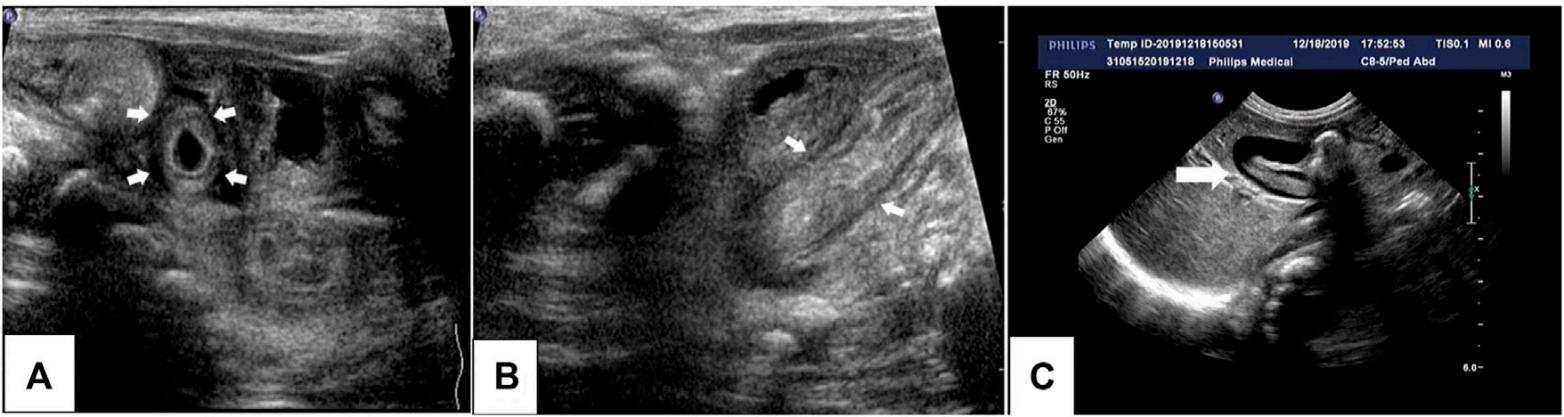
Ultrasonography of the proband’s abdomen. Thickening of the small intestine and colon, enhanced echogenicity of the mucous membrane and submucosa, and effusion in the colon are visible (A,B). Low-echogenicity area in the gallbladder (bile sludge) (C) can be seen, which disappeared the next day.
